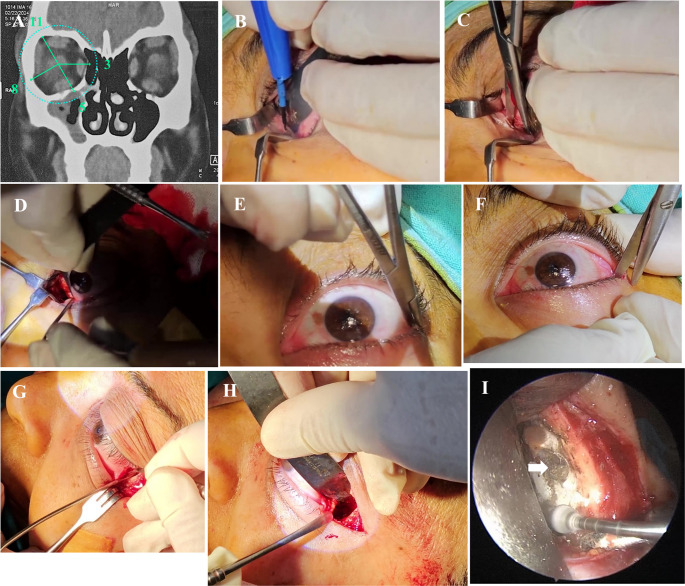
A 43-year-old woman developed exophthalmos and retro-orbital pain due to thyroid orbitopathy. Due to maxillary sinus infection and after interdisciplinary discussion, the decision was to use a precaruncular approach for medial orbital decompression between 3-5 o’clock, along with lateral canthotomy and cantholysis, and drilling of the zygomatic bone for lateral orbital decompression between 8-11 o’clock to keep the sterile orbit away from the contaminated nose. A) Coronal CT scan showing bilateral hypertrophied four recti muscles. B) Conjunctival incision with Colorado needle while protecting lacrimal puncta using retractors. C) Dissection to reach the subperiosteal plane using scissors. D) Outward fracture of the lamina papyracea using the freer. E and F) Crushing of the lateral canthal tendon, then cutting it with scissors. G and H) Release of periosteum using the freer to access the lateral subperiosteal plane. I) Drilling of the zygomatic bone in the lateral orbital wall was done until reaching the temporalis laterally (white arrow) using a diamond drill
